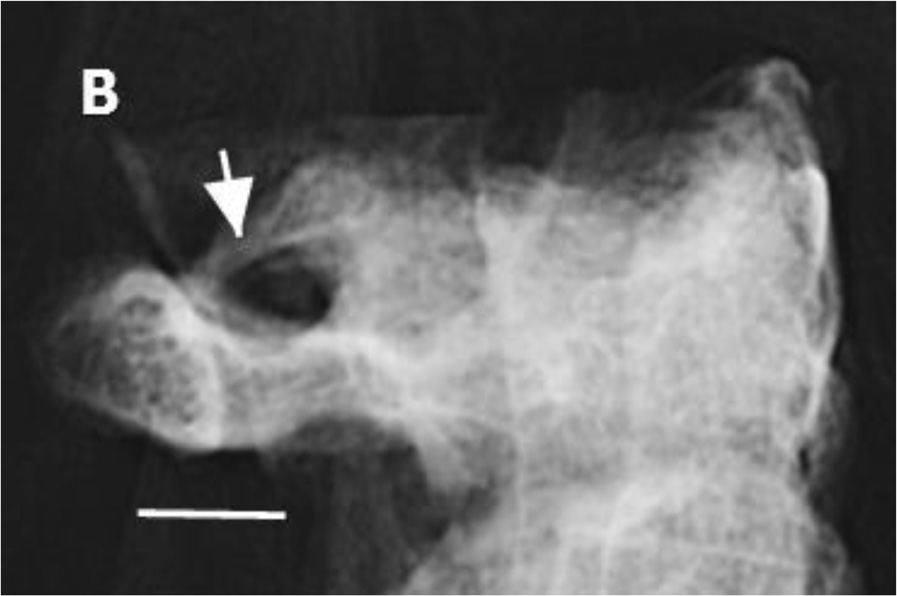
Lateral radiographic view of C1 and C2 vertebrae demonstrating complete ossification of membrane (*arrow*) forming an arcuate foramen. Linear bar represent 10 mm
